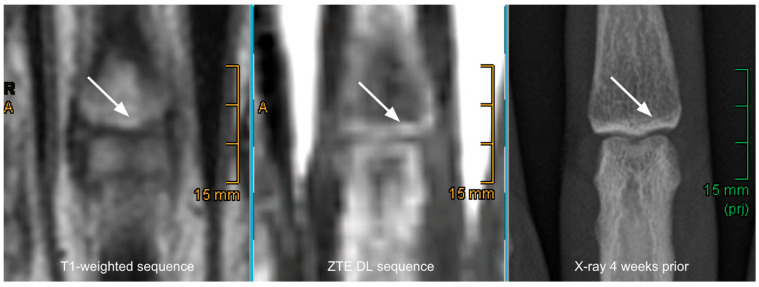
Sixty-year-old male with finger joint osteoarthritis, imaged with ZTE DL and T1-weighted MRI, with a corresponding radiograph obtained 4 weeks earlier. Potential subchondral sclerosis is better visualized at the third proximal interphalangeal joint with ZTE DL compared to T1-weighted MRI.
